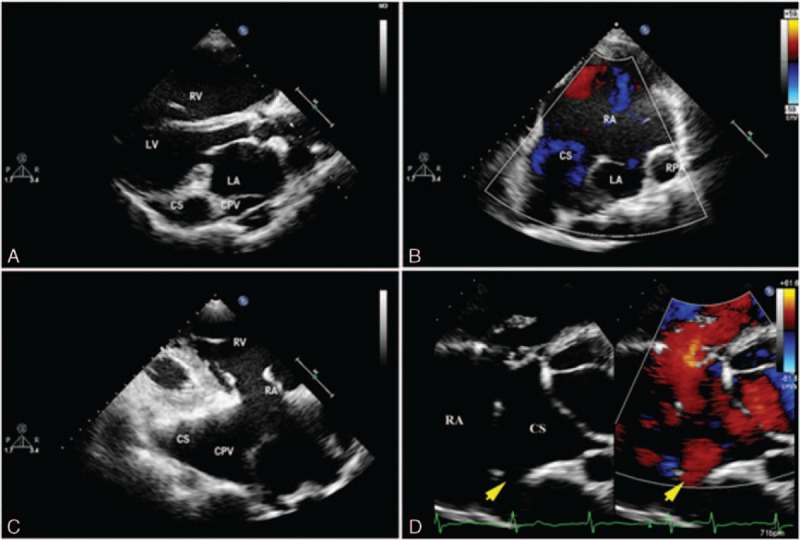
Multiple views showing the total anomalous pulmonary venous connection of a 53-year-old woman. (A) Common pulmonary vein (CPV) behind the left atrium and the enlarged coronary sinus (CS) in the left atrioventricular groove can be detected by parasternal long-axis view. (B) Anteromedial tilting bicaval view shows the CS drainage into the right atrium and atrial septal defect with right–left shunt flow. (C) CPV confluence to CS shown via the irregular right ventricular inflow tract view. (D) Flow spray to CS from CPV as shown by anteromedial tilting 4-chamber view.
